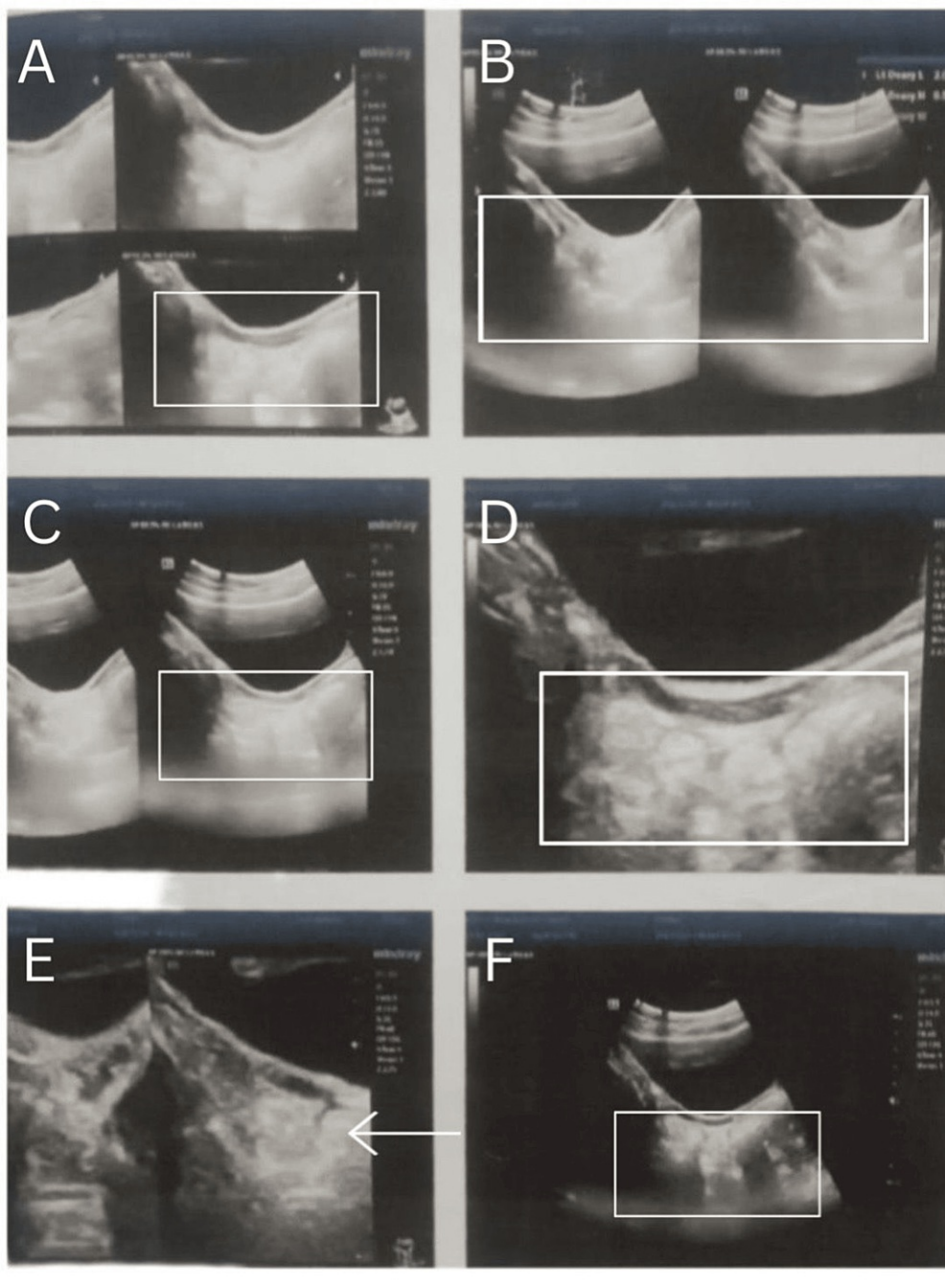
Ultrasonography report of the patient showing the hypoplastic uterus and streak ovaries A, B, and C panels depict the hypoplastic uterus, and D, E, and F panels show the streak ovaries.
